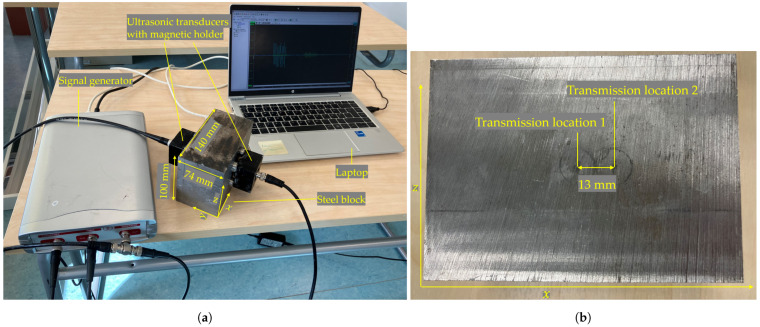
Experimental setup. (a) Measurement setup including ultrasonic transducers, a signal generator, and a laptop. (b) Transmission locations on the steel block.
